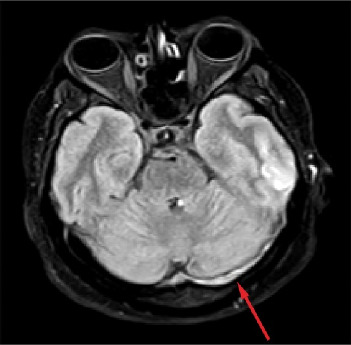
FLAIR MRI scan showing evidence of a cortical infarction in the left temporal lobe, visible as hyperintensity in the affected region and signal abnormality in the left transverse (lateral) sinus, consistent with sinus thrombosis, which is marked by an arrow.
